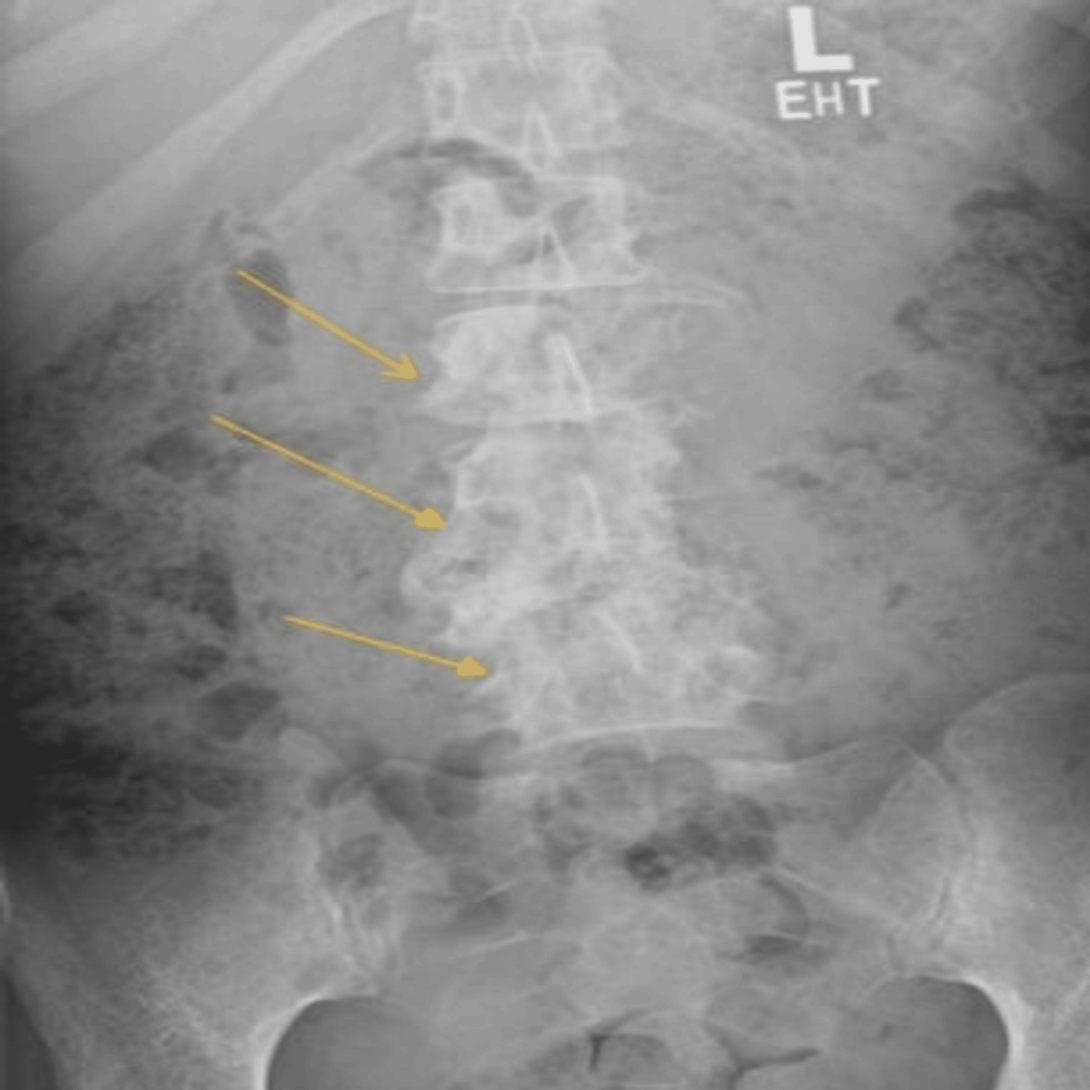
X-ray spine showing complete destruction of the left L2 and L4 vertebral bodies and a severe compression fracture at L4
